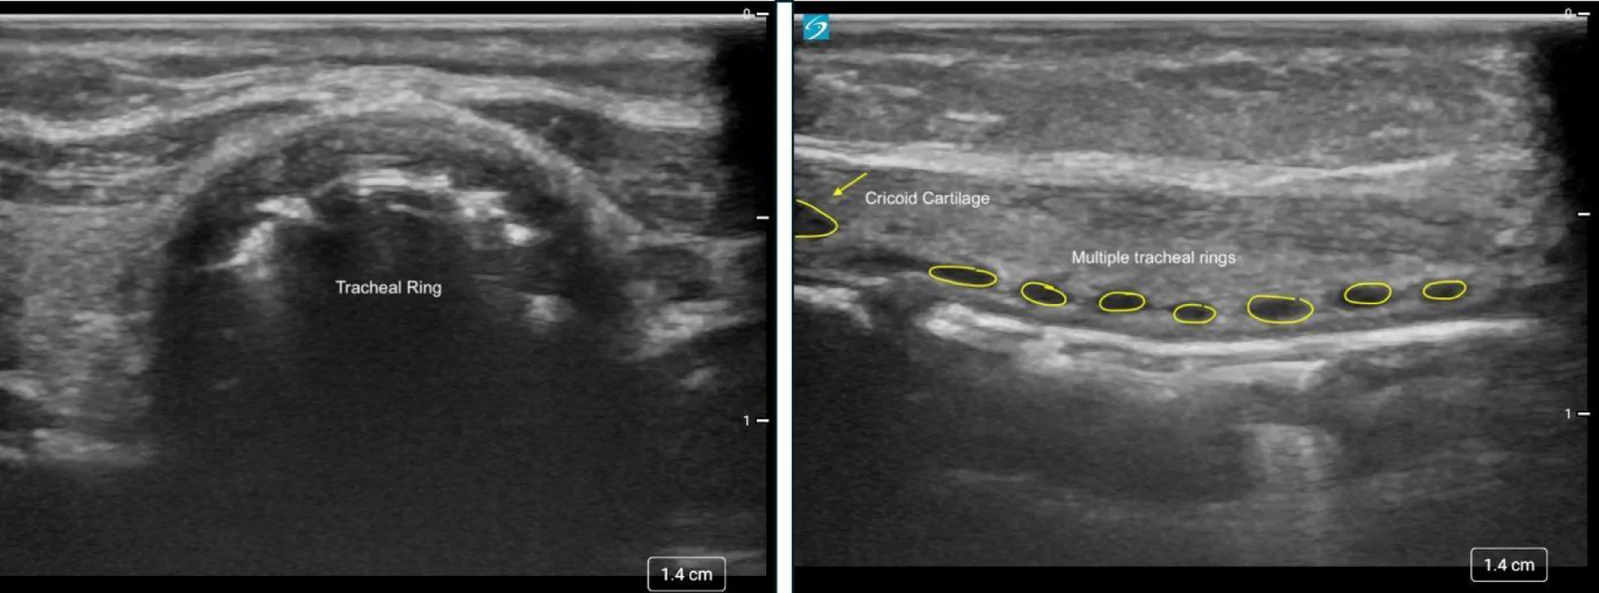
Transverse (left) and sagittal (right) ultrasound views of the area demonstrating the trachea, subglottic area, and cricothyroid membrane.
